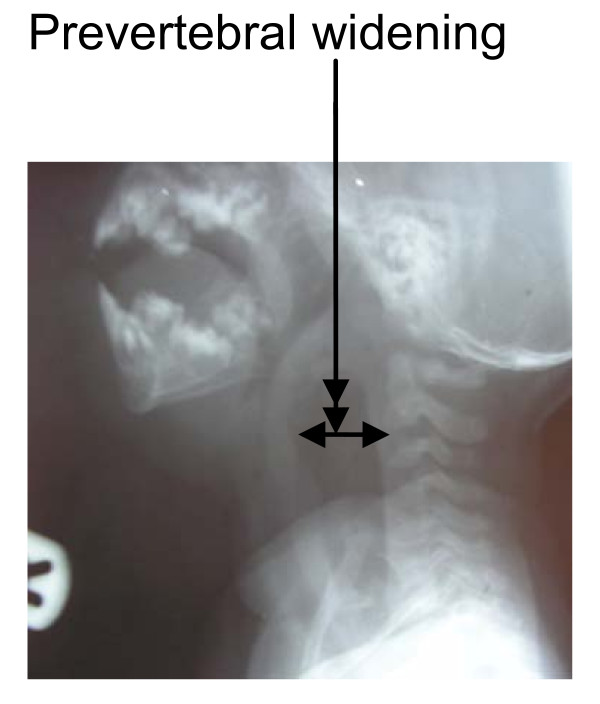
Lateral view X-ray showing the soft neck tissue and revealing widening of the prevertebral space containing areas of mixed opacity and lucency extending from the base of the skull to the level of the seventh cervical spine (C7), with the laryngeal air column almost obliterated, anterior displacement of the airway and straightening of the cervical spine.
